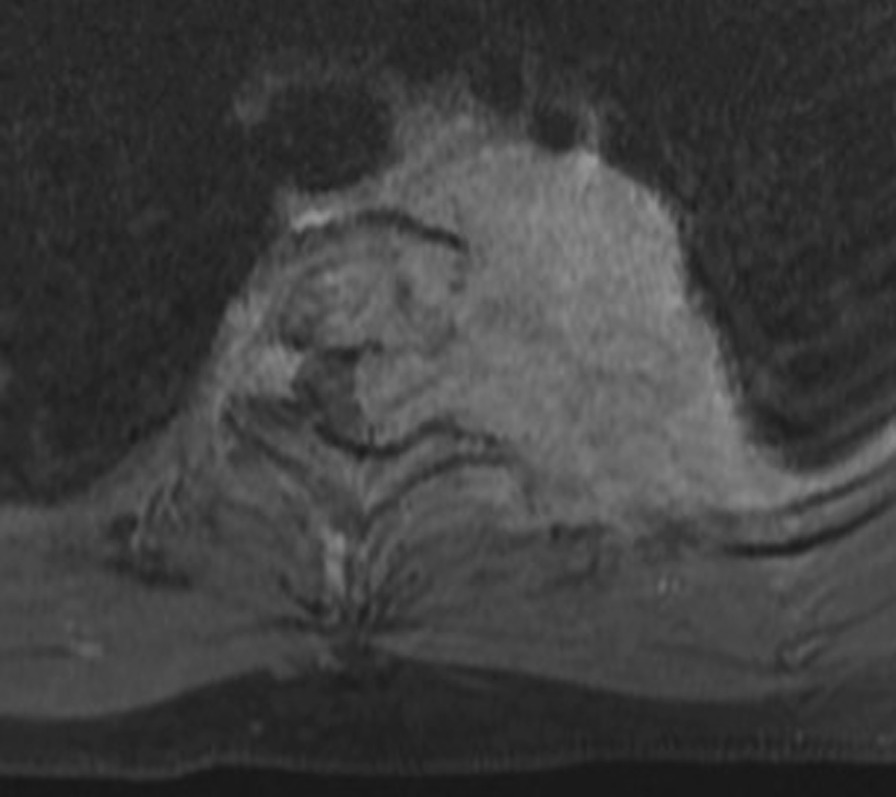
Magnetic resonance imaging (MRI) axial enhanced T1-weighted image at T2/T3 intervertebral level demonstrating avidly enhancing mass with neural foraminal extension and spinal cord displacement and compression. The mass is abutting the left subclavian artery and is eroding the left T2 transverse process, as well as the left third rib
